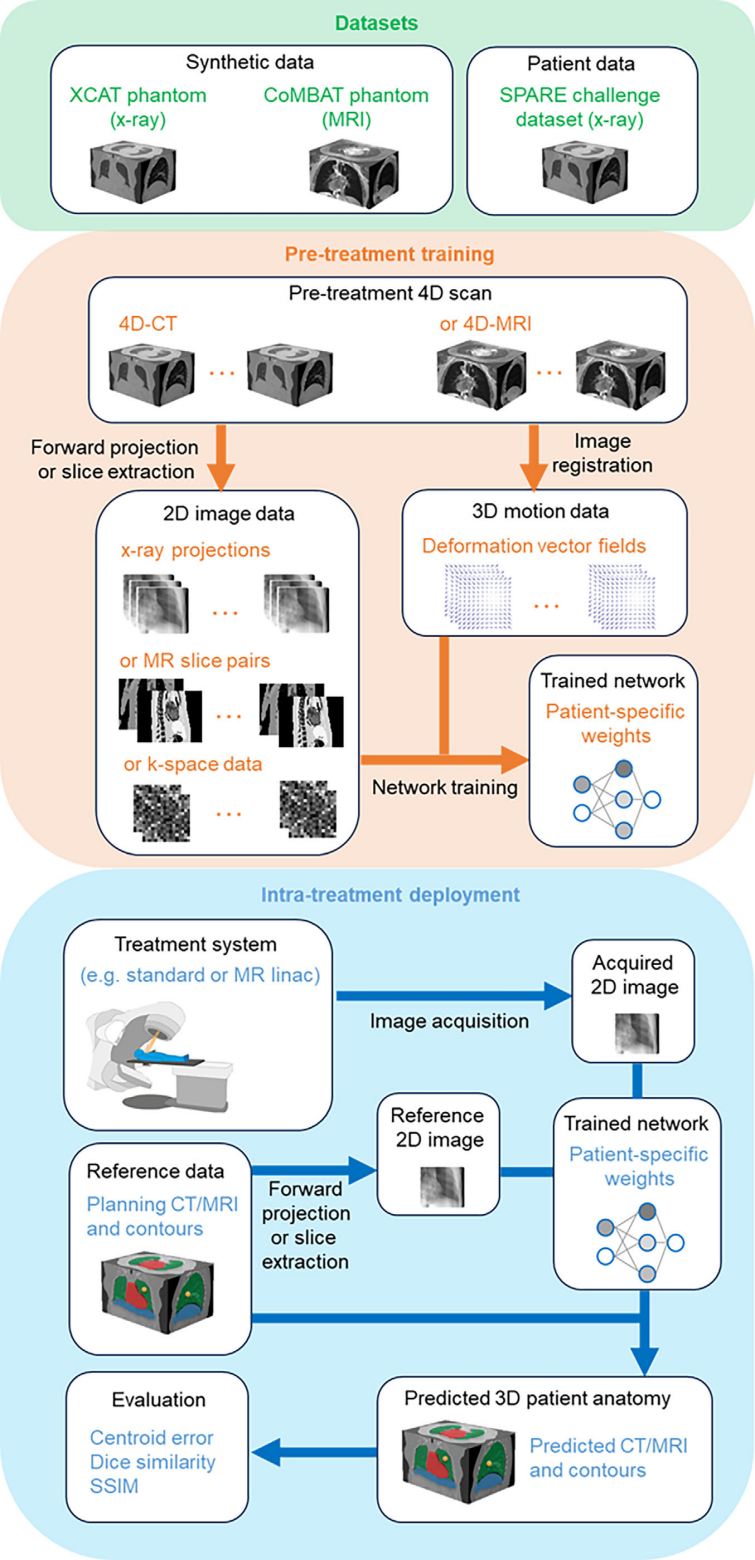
Overview of method developed and evaluated in this paper. XCAT means extended cardiac‐torso; CoMBAT means CT/MRI breathing XCAT; SPARE means sparse‐view reconstruction; CT means computed tomography; MRI means magnetic resonance image; linac means linear accelerator; and SSIM means structural similarity index measure.
